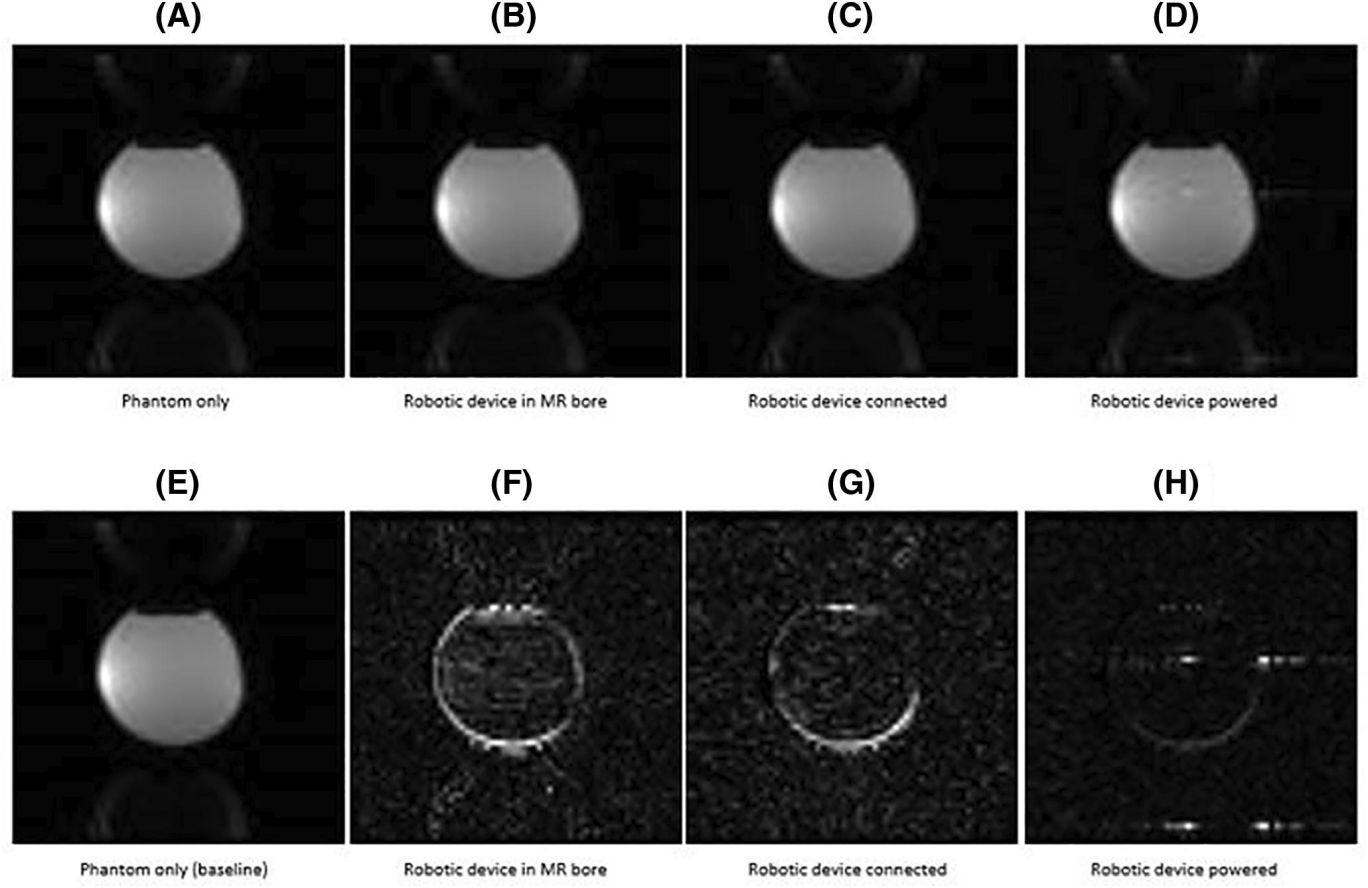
MR images (A–D) obtained using EPI sequence for different activation conditions of the robotic device and their corresponding differences (E–H) from the baseline image. EPI, Echo Planar Image; MR, magnetic resonance
